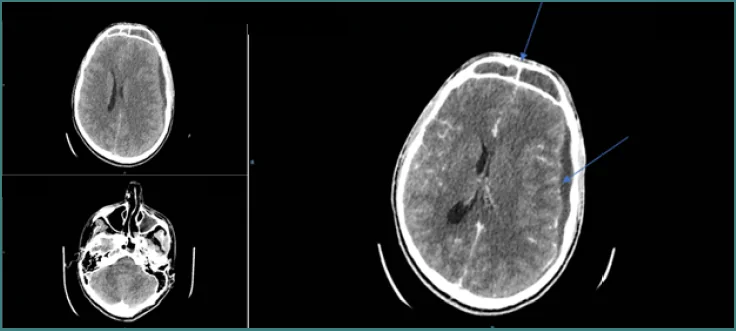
Initial cranial CT scan demonstrating a left fronto-temporo-parietal subdural collection (maximum thickness 13 mm) with mixed density and intralesional air bubbles, suggestive of anaerobic infection. An associated mass effect with mild midline shift is noted. Concomitant opacification of all paranasal sinuses is visible.
